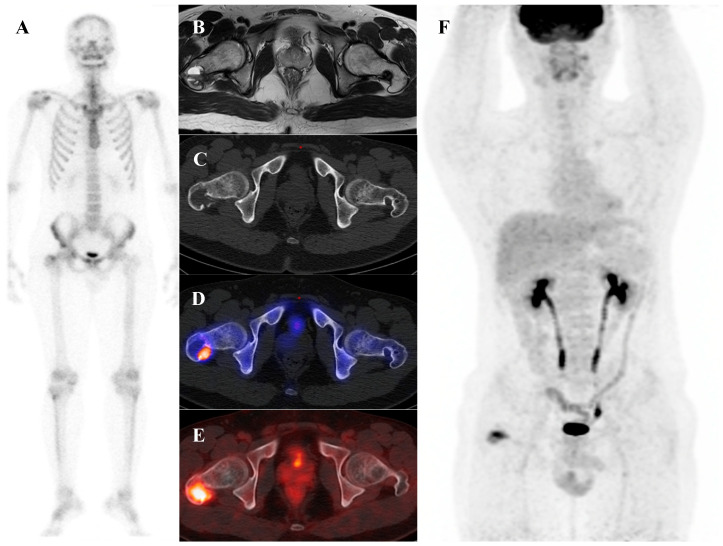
A 22-year-old male with chondroblastoma of the right femoral greater trochanter. (A) Bone scintigraphy shows focal uptake. (B) T2 fat-suppressed magnetic resonance imaging reveals an osteolytic mass with cortical disruption, extraosseous extension, fluid–fluid levels suggesting hemorrhage, nodular rim enhancement, and peritumoral edema, mimicking a malignant tumor with secondary aneurysmal bone cyst. (C,D) Bone single-photon emission tomography/computed tomography (CT) demonstrates a well-defined osteolytic lesion with a sclerotic rim and intense uptake at the site of extraosseous extension. (E,F) [^18^F]FDG positron emission tomography/CT shows intense uptake (SUVmax 9.8) at the lesion site. Surgical excision confirmed chondroblastoma with secondary aneurysmal bone cyst change.
